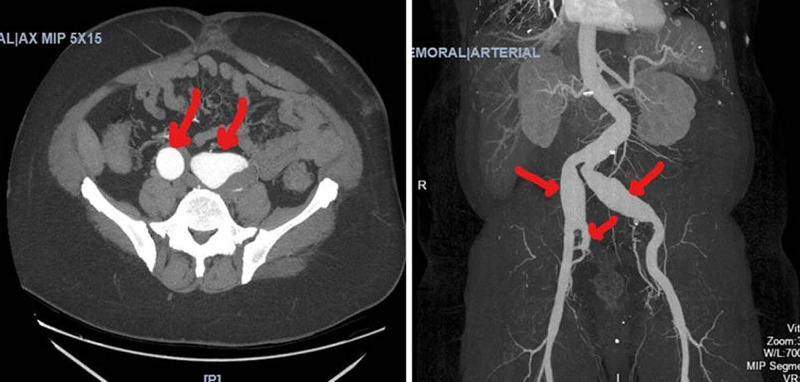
CT with contrast showing bilateral common iliac artery aneurysms and right internal iliac aneurysm.
